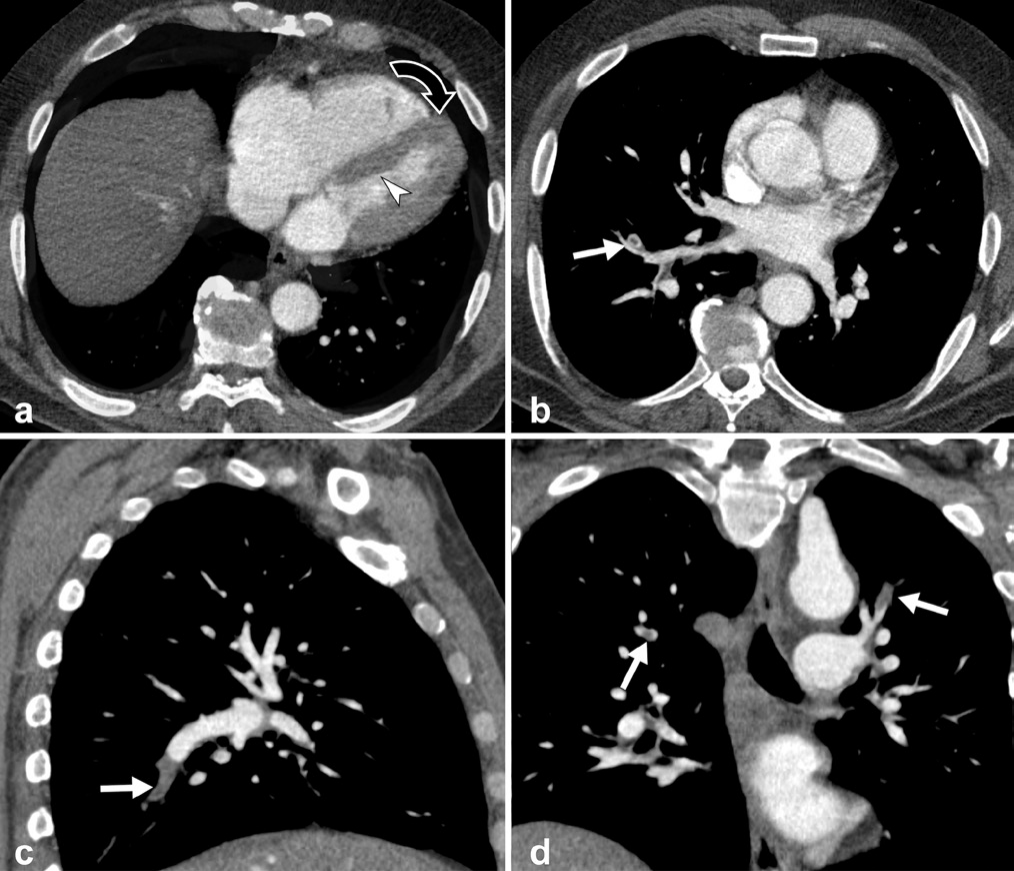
Contrast-enhanced CT scan during arterial-phase. (a) Lower chest CT image demonstrates CT signs of right heart overload: enlargement of right atrium and ventricle, cardiac axis clockwise rotation (curved arrow) and straightened interventricular septum (arrowhead). (b, c, d) Axial and MPR reformatted images showing bilateral segmental and subsegmental pulmonary embolism (arrows)
